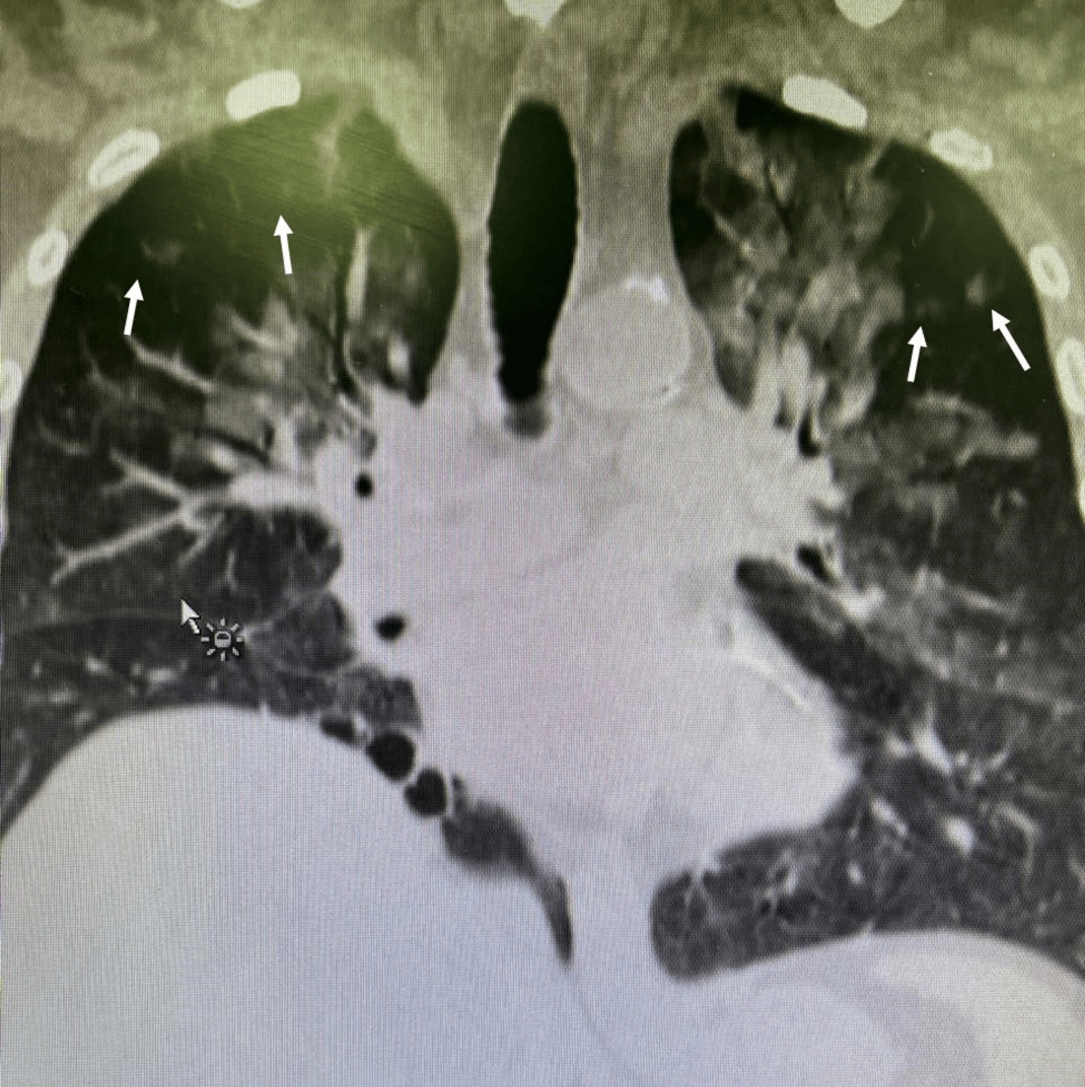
Chest X-ray showing resolution of both lungs after implementation of the treatment protocol (arrows).
